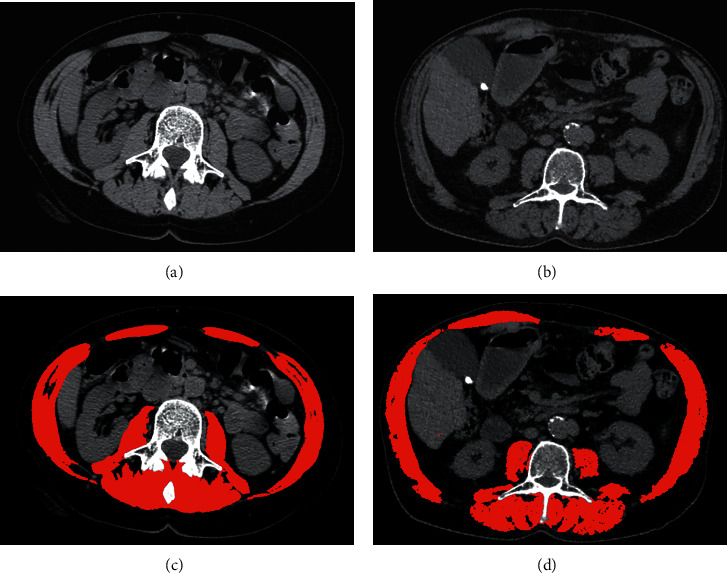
Computed tomography images of two different patients, one without cirrhosis (a) and one with cirrhosis (b). The patient without cirrhosis does not demonstrate imaging sign of sarcopenia using the public domain software (ImageJ) (c). The patient with cirrhosis demonstrates imaging sign of sarcopenia using the public domain software (ImageJ) (d).
